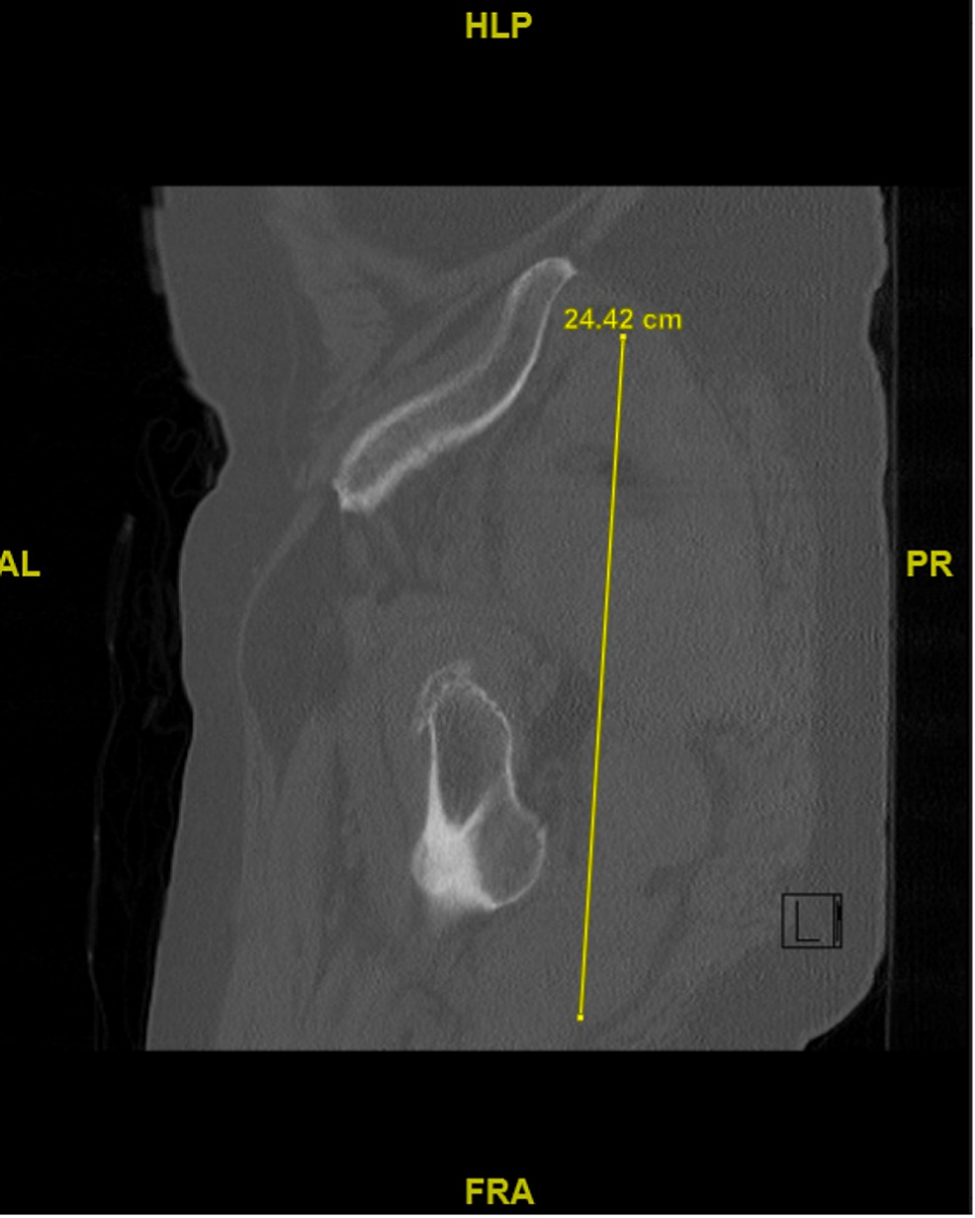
CT of pelvis showing a large intramuscular hematoma, extending inferiorly along the posterior aspect of the femur.
